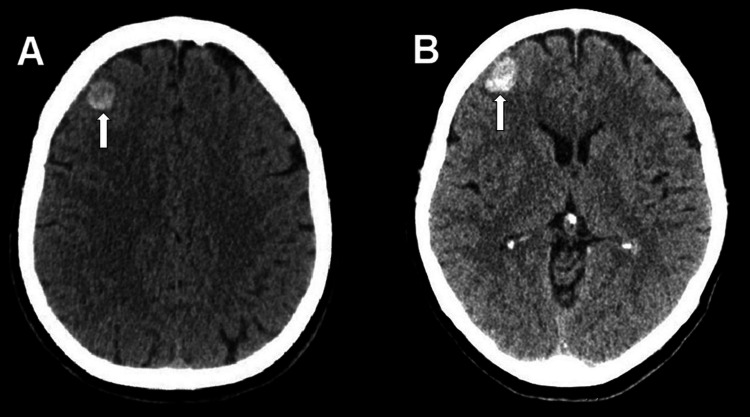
Axial CT images at admission in the emergency room (A) and six hours later (B) showing a round hemorrhagic lesion
